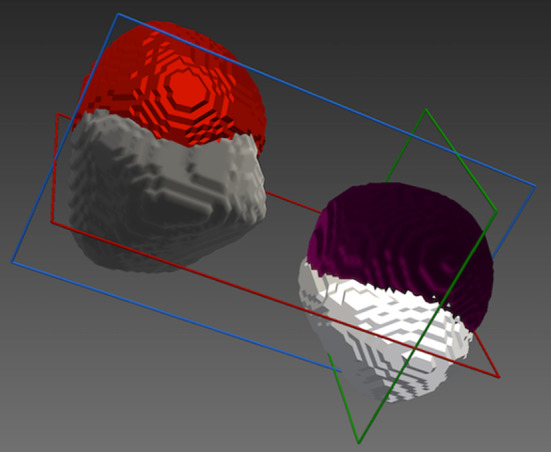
Segmentations of the tumour spheres obtained using registered volumetric ultrasound and 3D X-ray images. *Maroon/Red*: Segmentations of 3D X-ray images; *White/Grey*: Segmentations of reconstructed volumetric ultrasound images; tumour sphere 1: maroon/white; tumour sphere 2: red/grey
